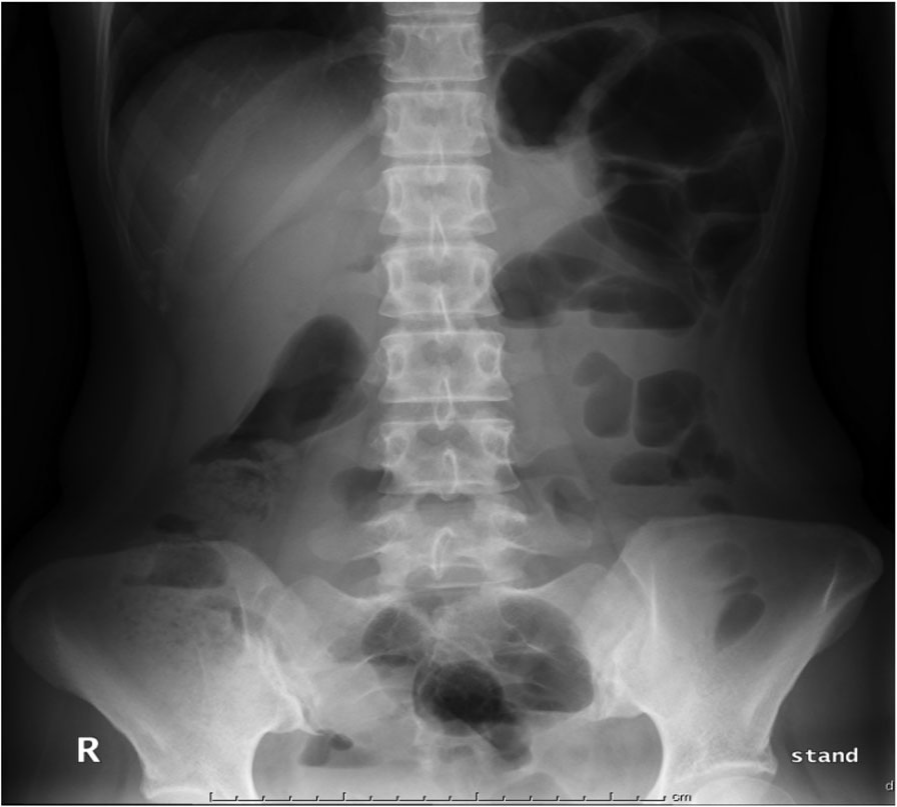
Abdominal x-ray findings: Niveau formation
